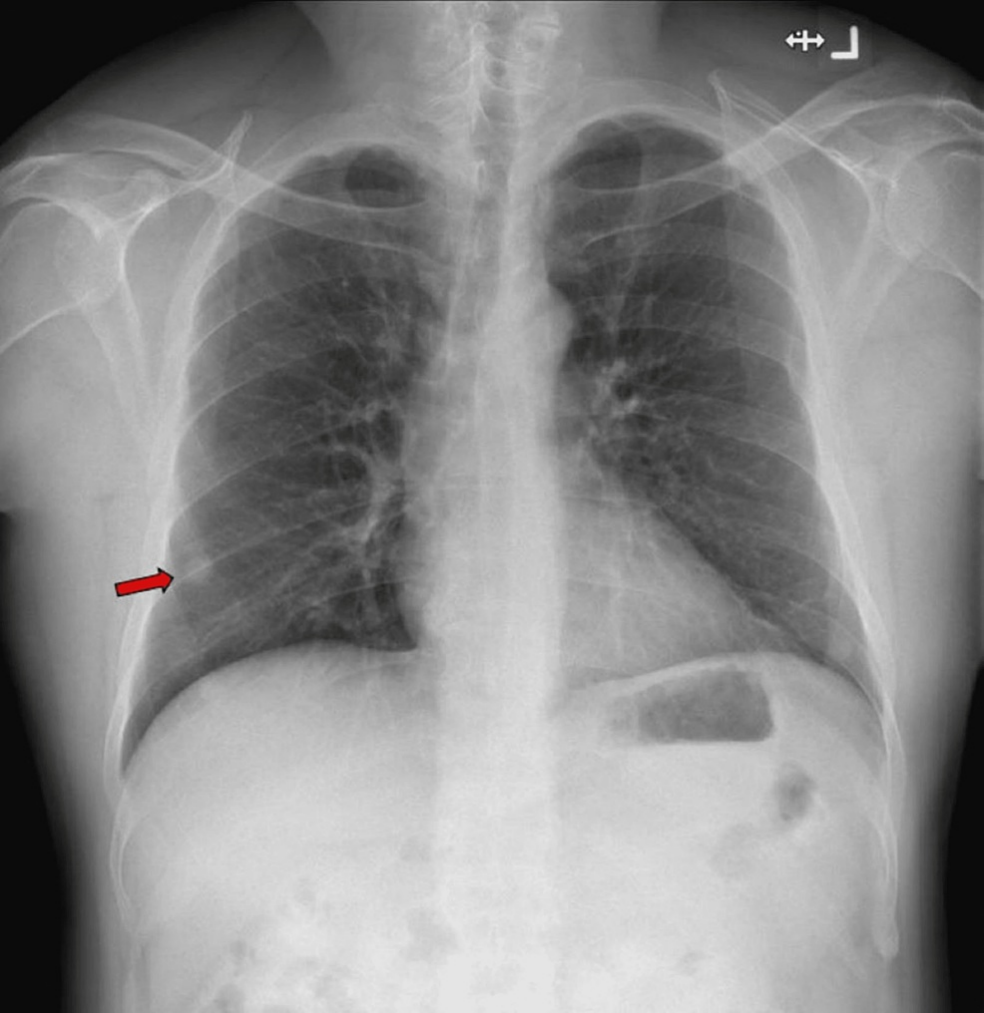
Chest radiograph on presentation to the emergency department showing a well-rounded opacity in the right middle zone.
